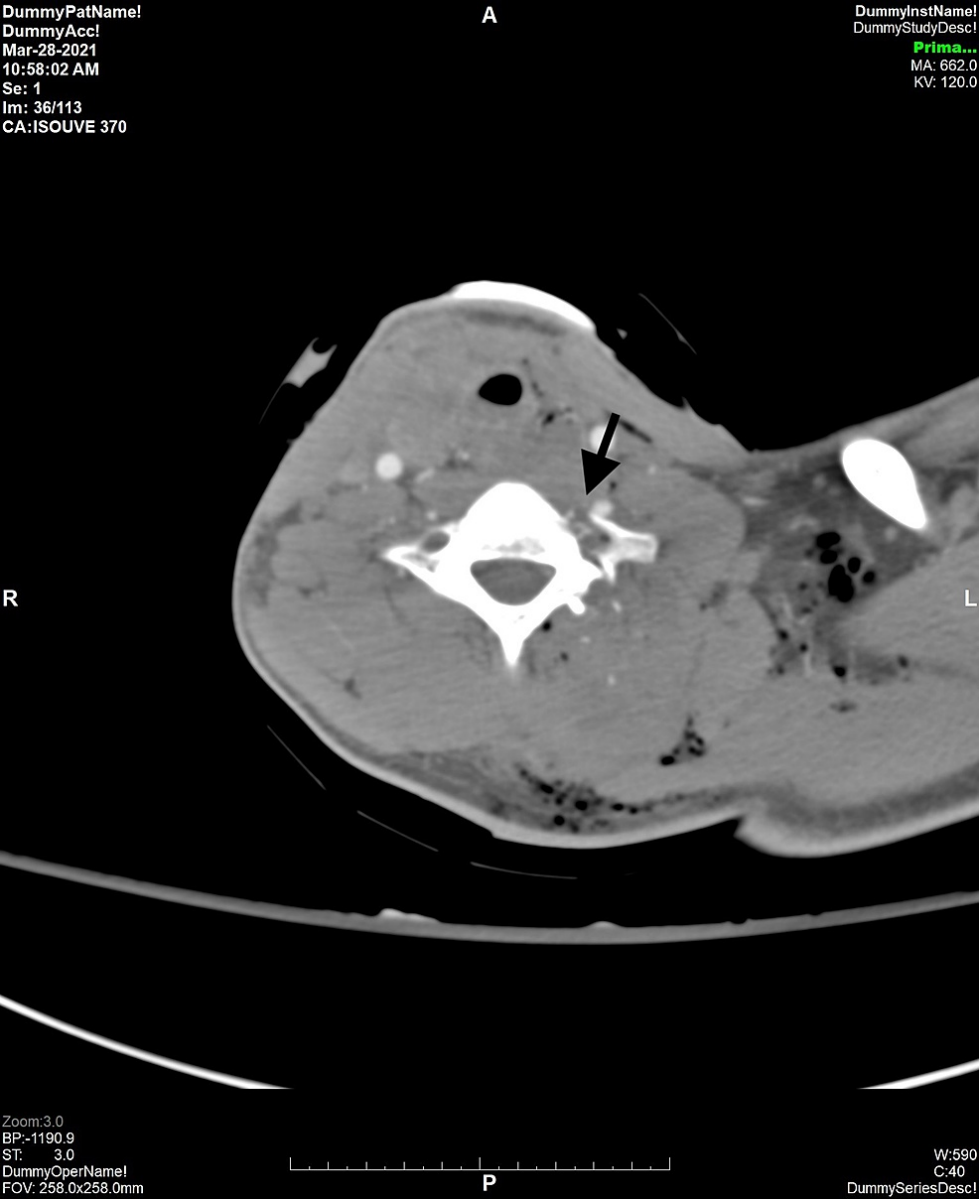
CT Image of the Cervical Spine (Axial View) Showing Fracture of the Left C7 Transverse Process (arrow)
